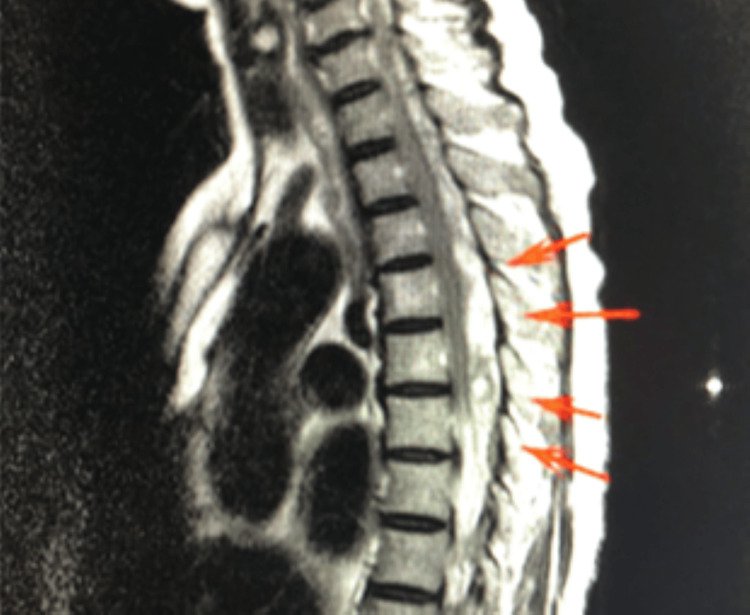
Spinal MRI confirming thoracic epidural hematoma from T3 to T6 with spinal cord compression. Arrows indicate the location of the hematoma.
